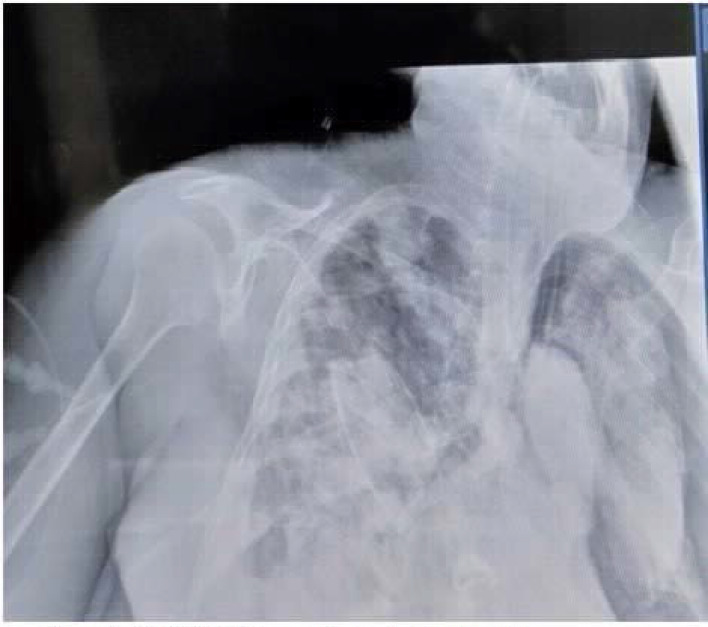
CASE 9: left pneumothorax in chest X-ray
